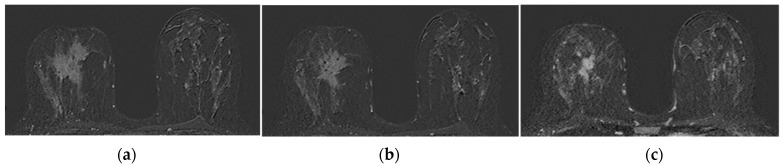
Partial pathological response (PR): large spiculated neoplastic lesion (4.5 cm) in right retroareolar (HER2 +) with moderate contralateral BPE in the pretreatment baseline image (a). Progressive reduction of lesion diameter is observed to 50% in step I MRI (b) and to 75% in step II MRI (c) without significant changes in BPE.
